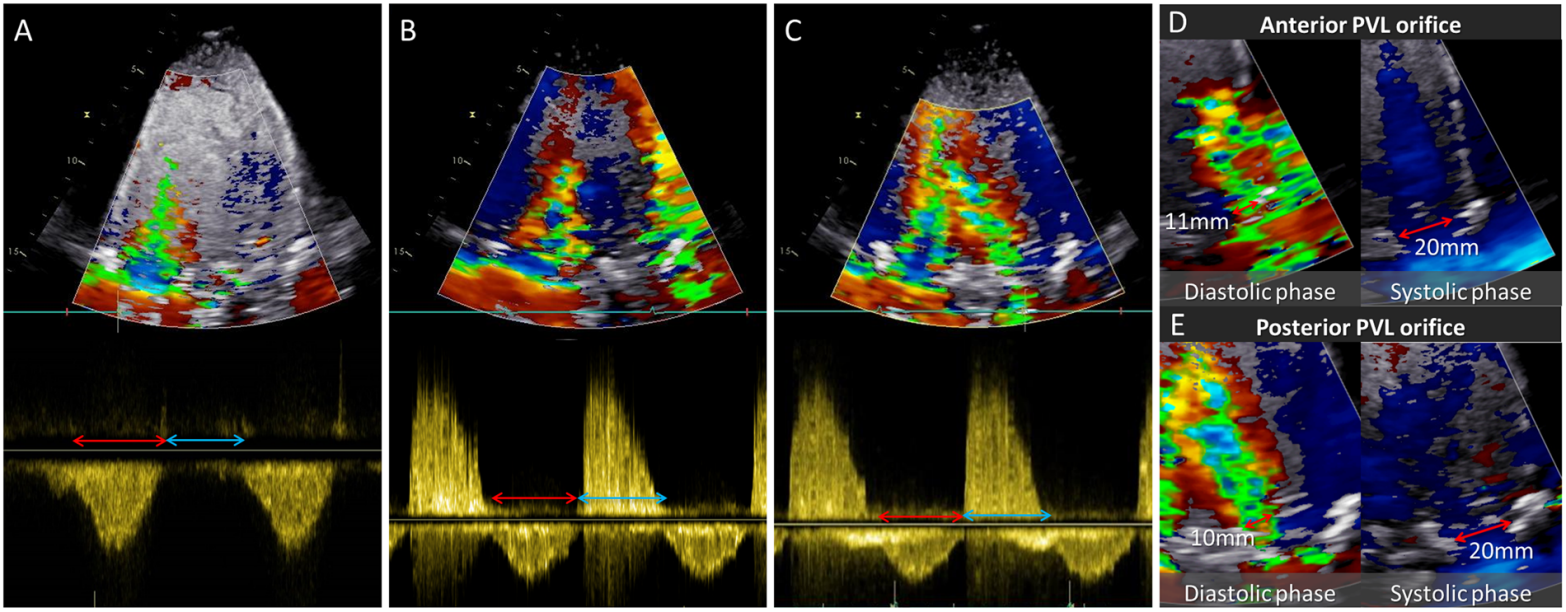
Color Doppler imaging, continuous Doppler echocardiography. (A–C) Color Doppler imaging and continuous wave (CW) Doppler echocardiography. Obvious regurgitant signal was observed in (B) and (C) and the interaction between the PVL jet and the transmitral flow was observed in (C). Red and blue arrows indicate systole and diastole, respectively. (D) and (E) Width of the PVL jet and outflow tract in anterior and posterior PVL case.
